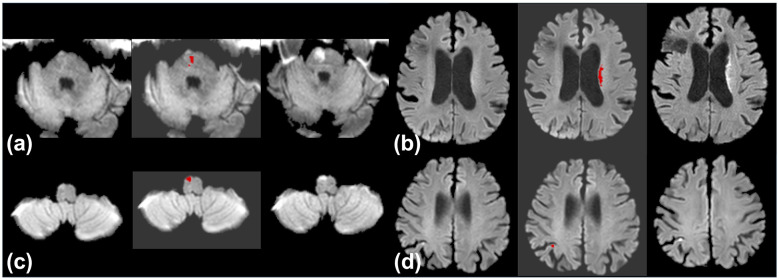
Representative cases of acute ischemic stroke lesions that were initially missed by most or all reviewers but correctly identified with AI assistance. (a) Pontine infarction not detected by any reviewer on initial MRI (15 h from last known well); identified by three reviewers with AI assistance. Follow-up MRI at 2 days confirmed the lesion. (b) Corona radiata infarction undetected by all reviewers on initial MRI (2 h); correctly identified by three with AI support. Confirmed on follow-up MRI at 4 days. (c) Medial medullary infarction not recognized by any reviewer at baseline MRI (3 h); identified by three with AI assistance. Confirmed on follow-up at 2 days. (d) Subtle parietal cortex infarction missed by four reviewers on initial MRI (2 h); correctly detected with AI. Follow-up at 4 days confirmed the lesion.
